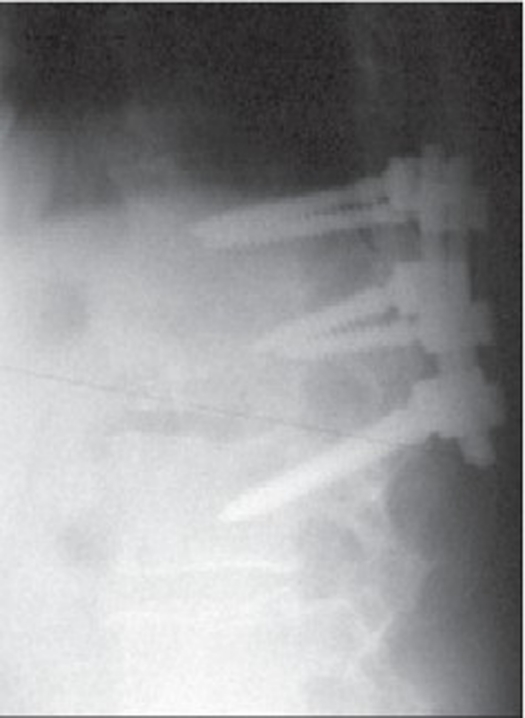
Lateral roentgenogram of the same patient 31 months postoperatively showing Calcibon incorporation
